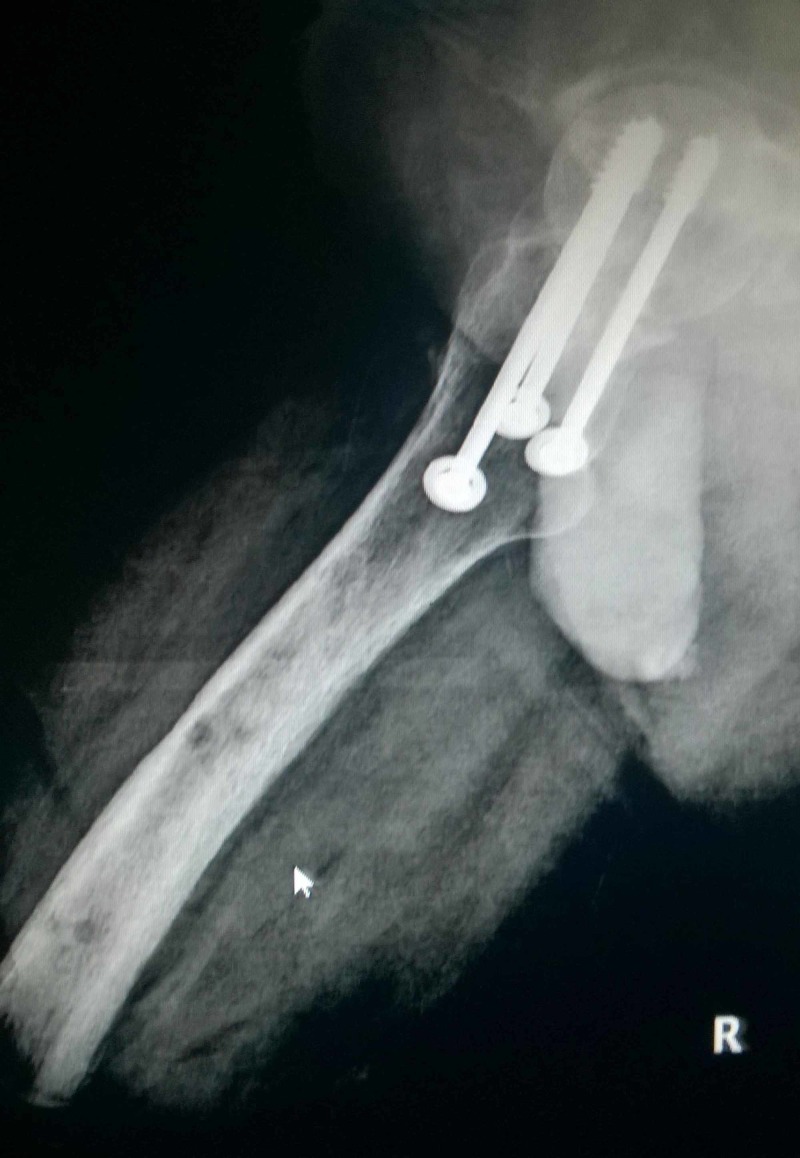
One and half years follow up lateral radiograph of patient A
